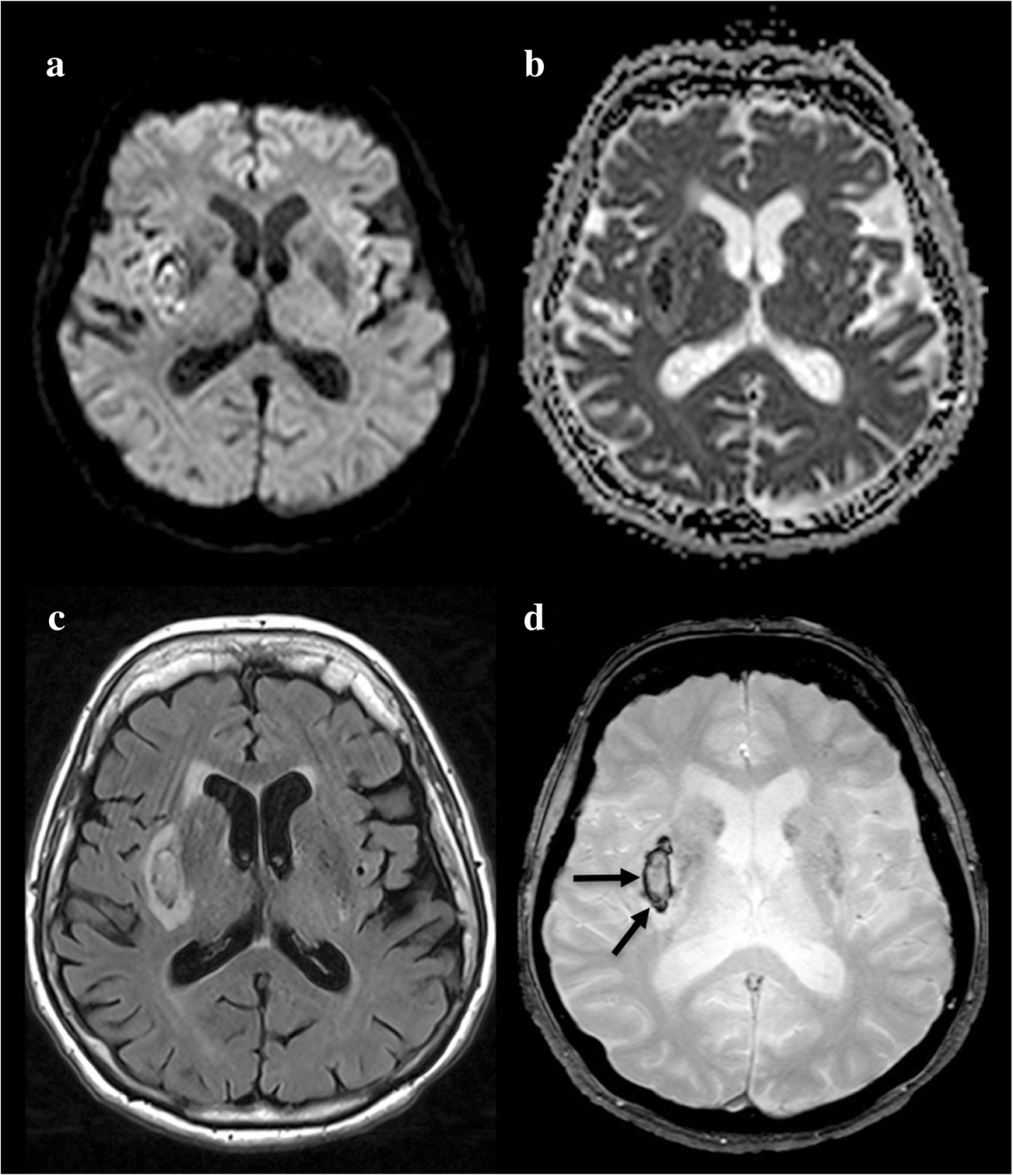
A 48-year-old woman with intracerebral haemorrhage presenting with left hemiplegia and left homonymous hemianopsia. DWI shows heterogeneous signal abnormalities involving the right lenticular nucleus on b1000 (a), apparent diffusion coefficient (ADC) (b) and fluid-attenuated inversion recovery (FLAIR) (c). The lesion appears as a peripheral hypointensity on T2 with gradient recalled echo weighted imaging (T2-GRE) (d, arrows)
